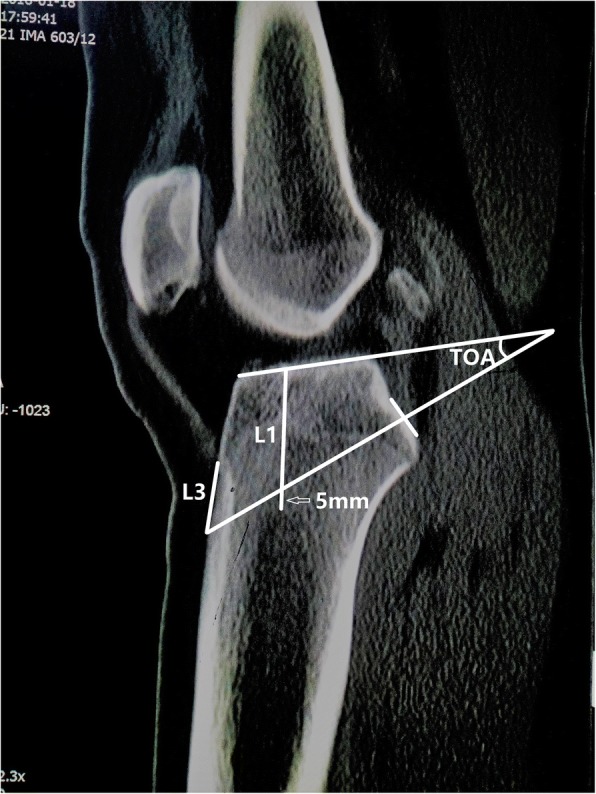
CT sagittal section: L3 was measured from anterior tunnel aperture to tibial tuberosity (lowest edge of patellar ligament attachment)
